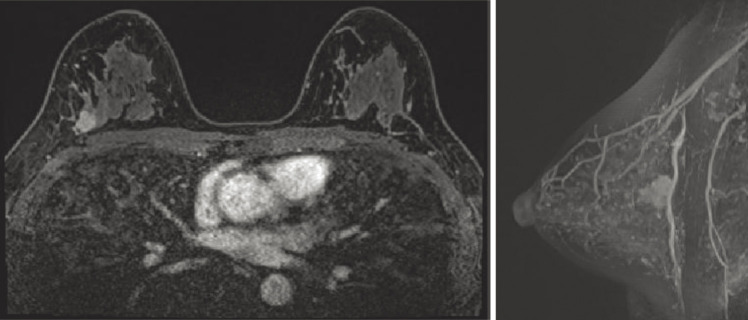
Contrast-enhanced magnetic resonance imaging showed an enhanced irregular mass in the right breast.
